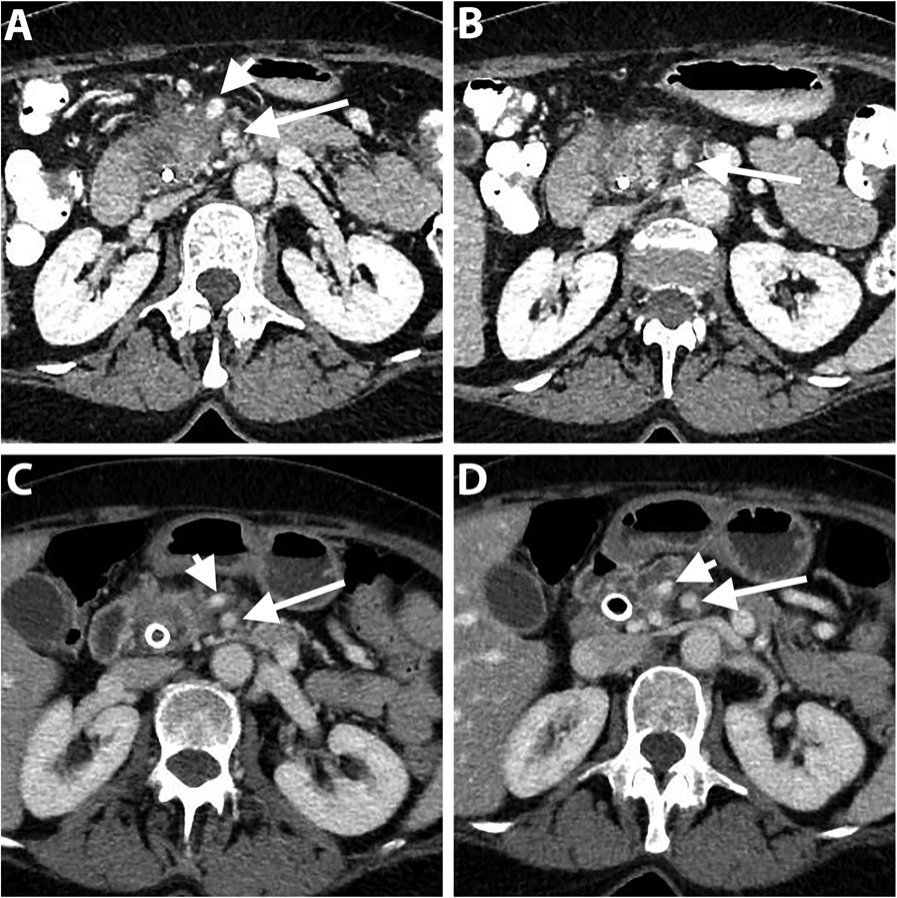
A 62-year-old woman with PDAC of the head. Axial portal venous phase CT images (a and b) show an ill-defined hypoattenuating mass encasing SMA (long arrow). SMV demonstrates a teardrop sign (short arrow) and is completely obliterated at a higher level (not shown). Axial portal venous phase CT images (c and d) obtained 2 months after neoadjuvant therapy show interval reduction of the tumor size and tumor contact with SMA (long arrow) and SMV (short arrow). The patient underwent pancreaticoduodenectomy and pathology revealed no residual invasion to SMA
